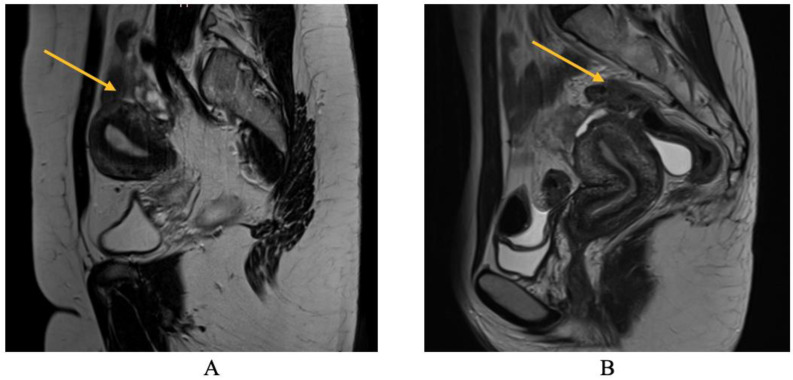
MRI aspect on T2 sagittal (A,B): recto-sigmoidian endometriosis nodule adherent to the uterine fundus via adenomyosis lesion.
